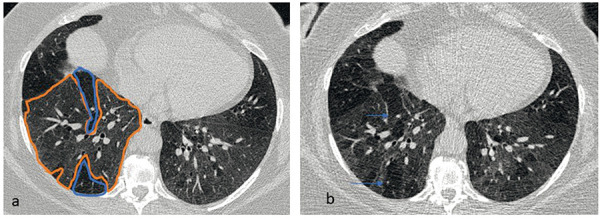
Noncontrast HR-CT obtained at inspiration in HP. Axial CT images at the level of the lower lobes. a: Initial findings; b: Low dose HR-CT after 2 years. a, b: Nearly identical manifestation of diffuse ground-glass opacities (a highlighted in orange on the right) – interrupted by hypodense (a highlighted in blue on the right) areas of air trapping. The persistence of groundglass opacities and air trapping over 2 years is consistent with fine fibrosis and chronic bronchiolitis. Note: Reduced vessel caliber in the air trapping areas due to secondary mosaic perfusion (b indicated by arrows on the right).
